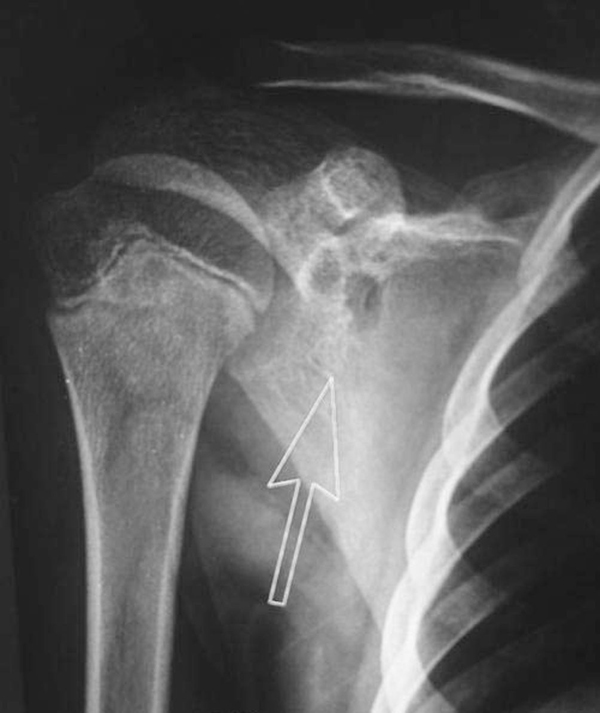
Anteroposterior (AP) radiograph of the shoulder showing two well defined lytic destructive lesions involving the glenoid margin suggestive of cystic tuberculosis.
